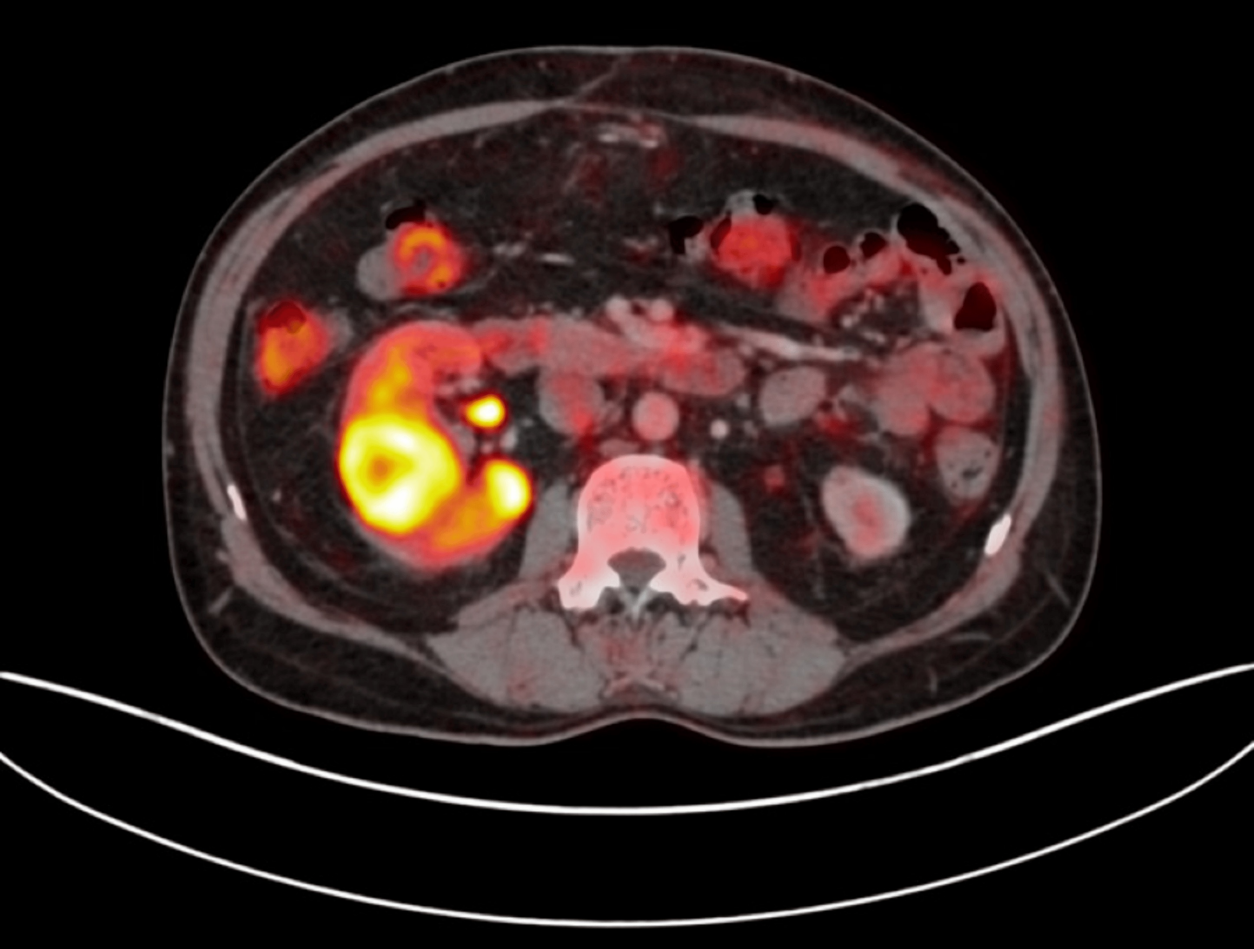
Transaxial images of the abdomen showing FDG concentration in heterogeneously hypoenhancing lesions in the right kidney
